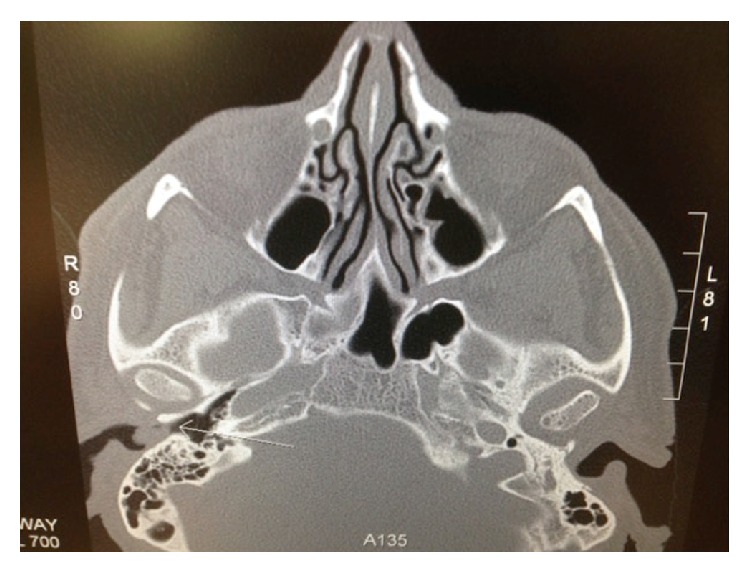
CT scan of the temporal bones, axial view, showing 1 cm polypoid soft tissue mass (arrow) in the external auditory canal, with no underlying exostosis or osteoma identified.
